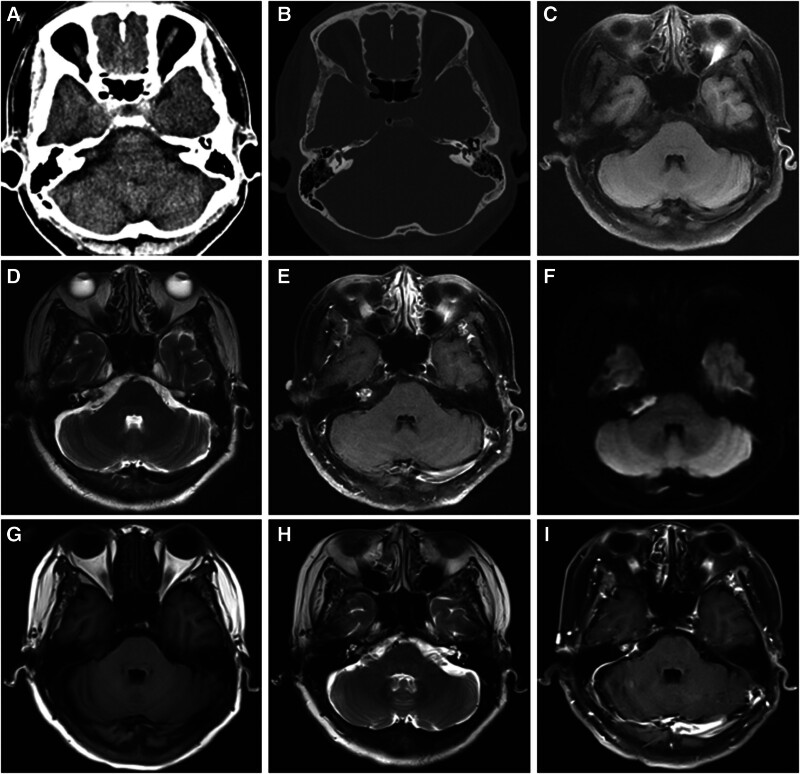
Preoperative images (A–F) and postoperative images at 1 year (G–I) were presented. Head axial CT images showed a mixed density expansive lesion of the right CPA region (A) and there was no significant bone damage to the internal auditory meatus on the affected side (B). T1- and T2-weighted imaging demonstrated a cyst and solid lesion with heterogeneous signals located in the right CPA region (C, D). T1-weighted gadolinium-enhanced MRI exhibited obvious mass-like enhancement in the solid part and hypointensity in the cystic part (E), while DWI showed an opposite signal (F). An anomalous signal was detected in the right internal auditory canal, presenting with slightly hypointense signal on T1 (G) and a mixed slightly hyperintense signal on T2 (H), as well as uneven enhancement on enhanced scan (I). CPA = cerebellopontine angle, CT = computed tomography, DWI = diffusion-weighted imaging, MRI = magnetic resonance imaging.
